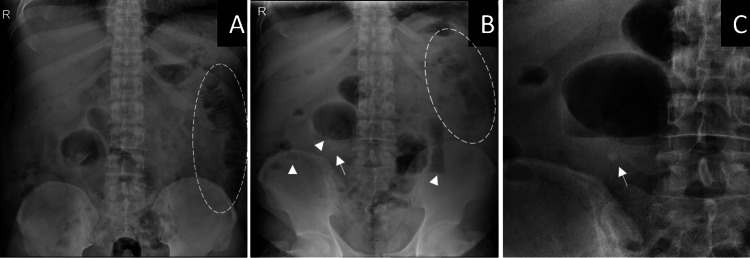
Abdominal radiograph. Supine (A) and erect (B) X-ray views show signs of small bowel obstruction including dilated small bowels with visible valvulae conniventes (dashed circles) and few air fluid levels (arrow heads). Small rounded calcified density is seen in the right lower quadrant (arrow) which is better visualized in the magnified view (C).
